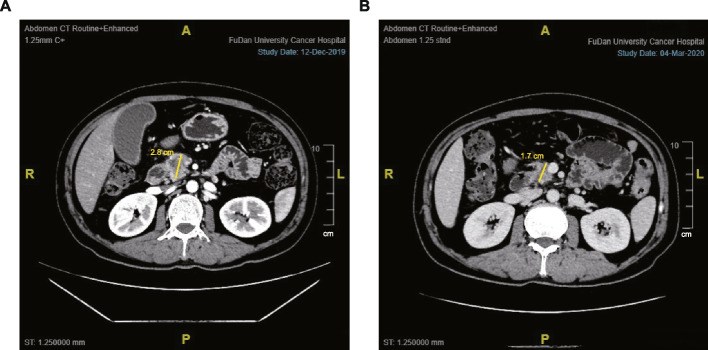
CT images of a patient who received 3 cycles of nab-paclitaxel plus gemcitabine regimen NACT, and the tumor was evaluated as partial response after the treatment. A CT image before NACT. B CT image after NACT. Abbreviation: NACT, neoadjuvant chemotherapy
